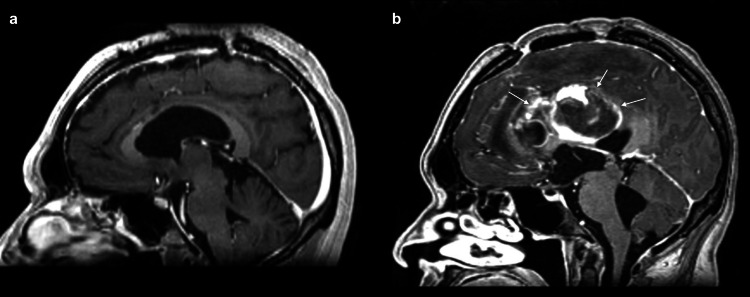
Sagittal T1-weighted brain MRI with gadolinium contrast enhancement obtained at two time points in a patient undergoing stereotactic radiosurgical callosotomy (a) Shows baseline images acquired three months before radiosurgery, demonstrating a corpus callosum without signal abnormalities. (b) Depicts the follow-up MRI obtained 18 months after treatment, revealing focal contrast enhancement and T1 signal hyperintensity within the irradiated segment of the anterior corpus callosum, indicated by the white arrows, consistent with post-radiosurgical changes. These findings suggest localized disruption of white matter integrity in the target region, in accordance with the planned therapeutic goal.
